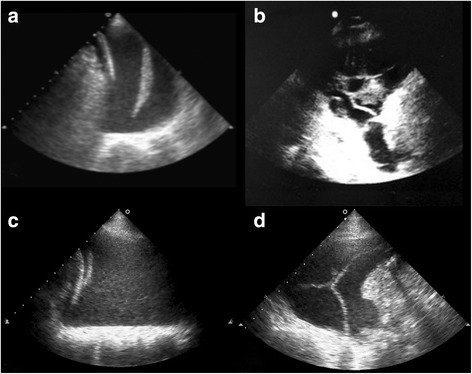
Different types of pleural effusion on ultrasound scan: a exudate, b empyema, c haemothorax, d complex septation pleural effusion
